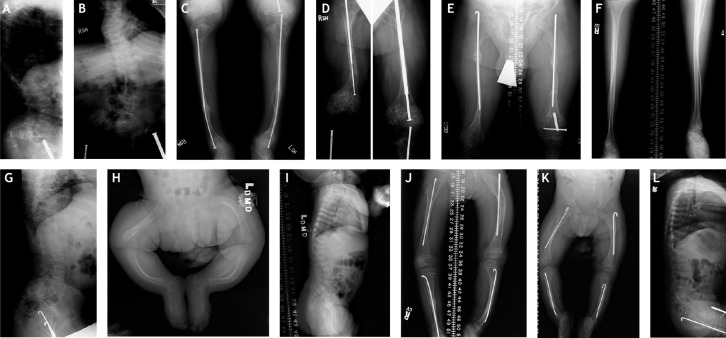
X-rays of patients 1, 2, 3 and 4 with OI show phenotypic variability associated with the same glycine substitution with identical or different bulkier amino acids. (A-D) Radiographs of patient 1, taken at 13 years 2 months. (E-G) Radiographs of patient 2, taken at 15.5 years. (H,I) Radiographs of patient 3, taken at 5.5 months. (J-L) Radiographs of patient 4, taken at 5 years 7 months (J), 2 years 10 months (K) and at 4 years (L).All radiographs were taken at the NIH Clinical Center following internal review board-approved protocols and none have previously been published.
